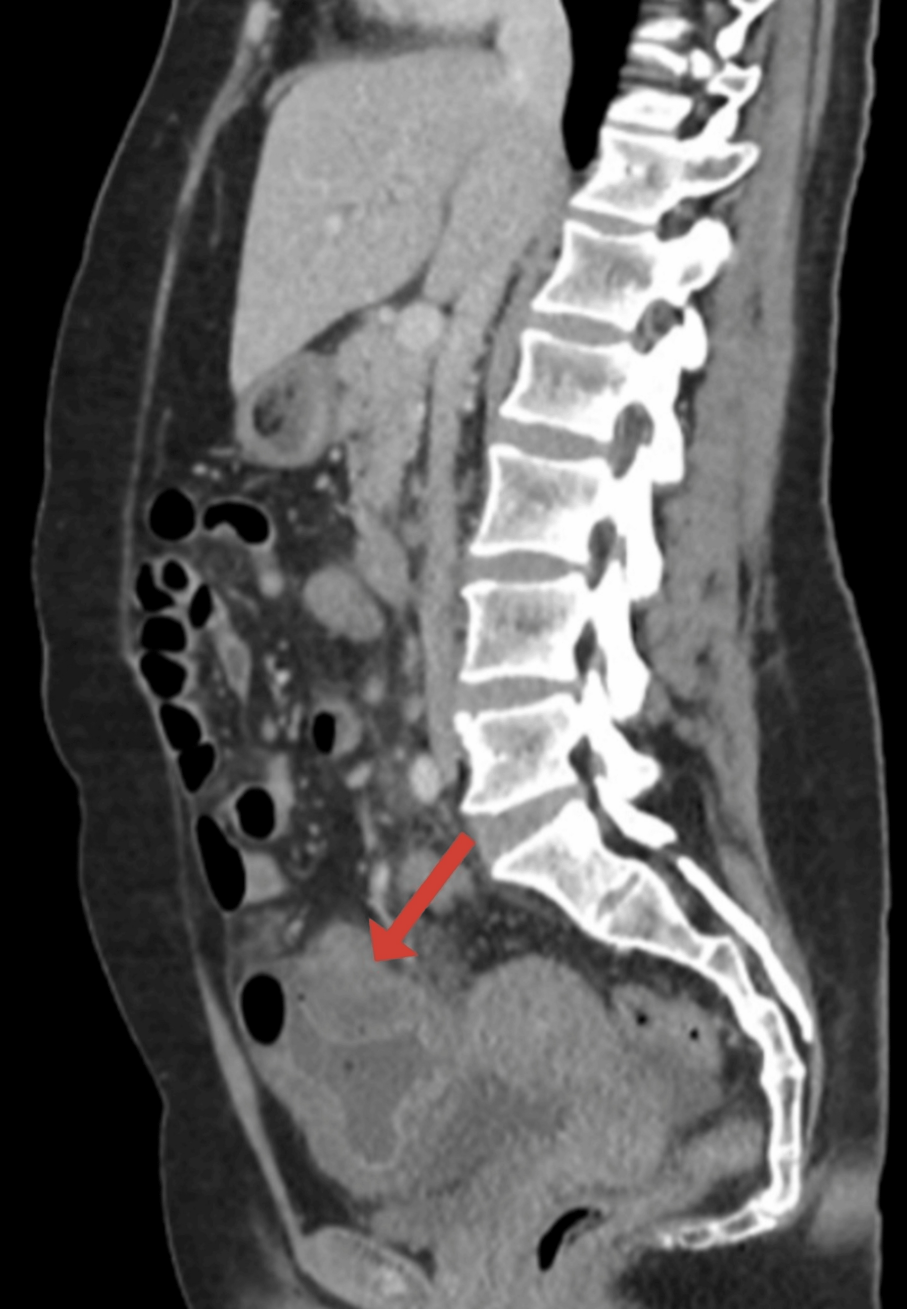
Contrast-enhanced CT of the abdomen (sagittal view) Segmental involvement of the cecum with wall thickening and layered enhancement (red arrow), without evidence of perforation.
